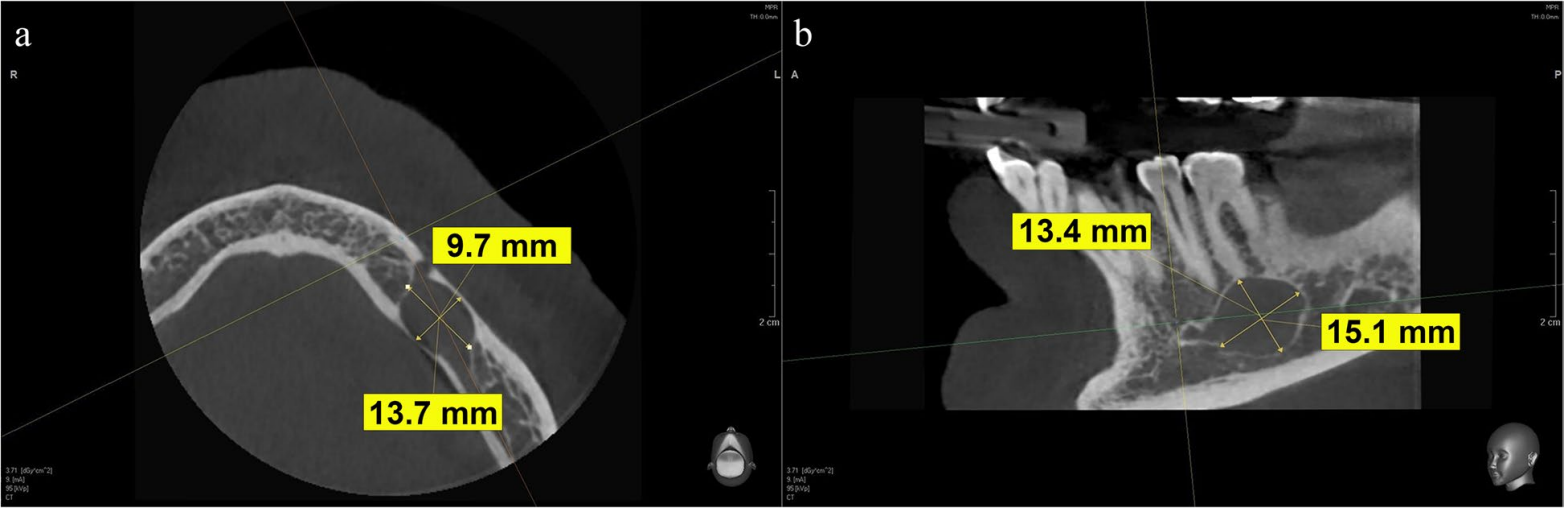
A 5 × 8 cm CBCT of the left mandible. a Axial view. b Lateral view
